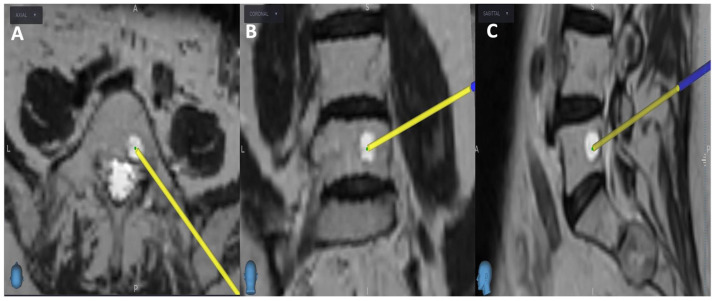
MRI scan; the yellow projection line displays the targeted biopsy site for the needle biopsy procedure: (A) Axial view; (B) Coronal view; (C) Sagittal view.
